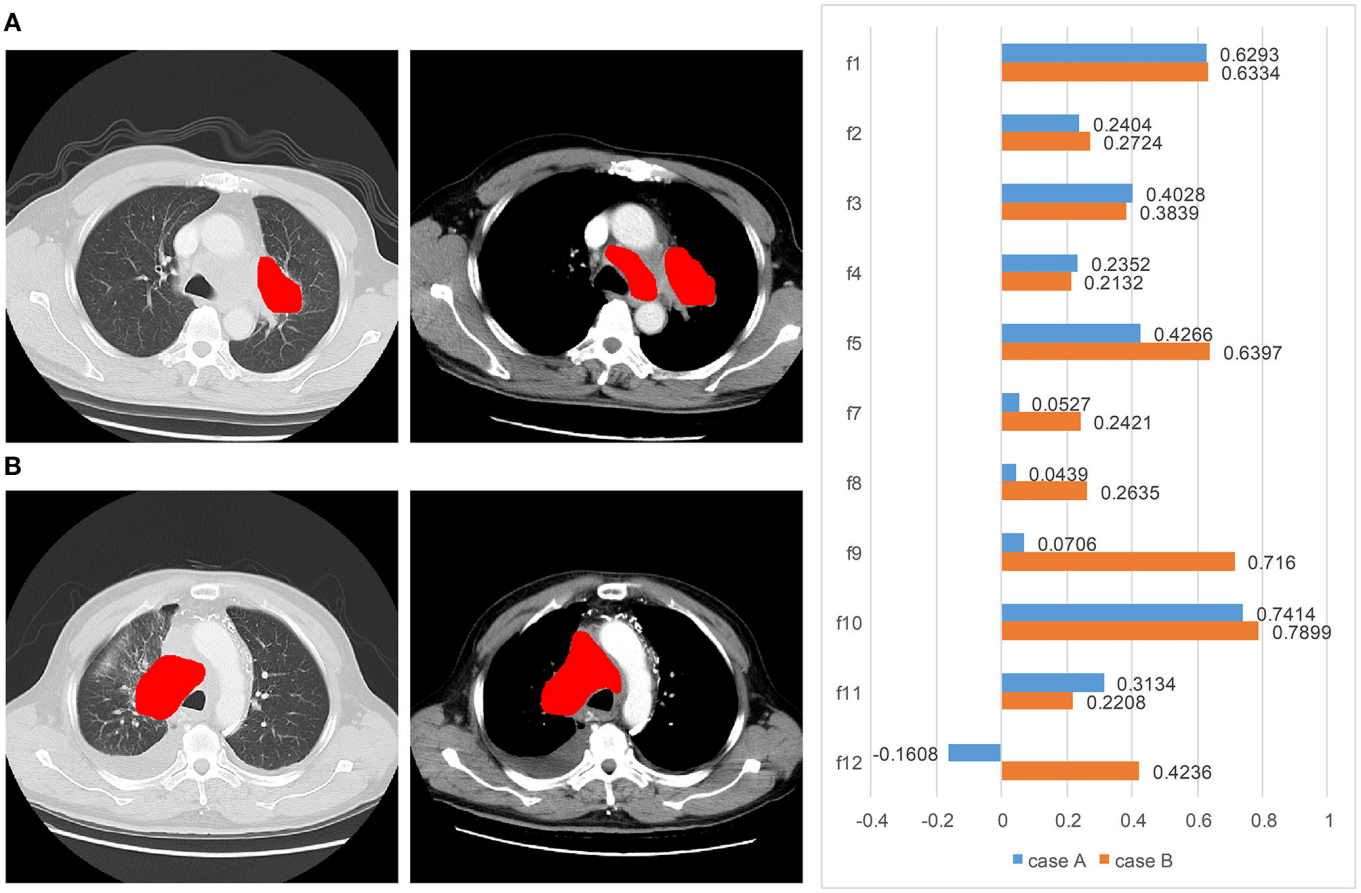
Two typical cases from the visual analysis and our method: (A) a patient (male, age = 62 years, limited stage) with PFS of 90 days. Our model correctly predicted it with a high risk of 157.0, but the visual and clinical prognosis was good. (B) A patient (male, age = 67 years, extensive stage) with PFS of 431 days. Our model correctly predicted it with a low risk of 55.0, but the visual and clinical prognosis was poor. From left to right are the lung window CT, enhanced mediastinal window CT, and the feature weights, respectively.
